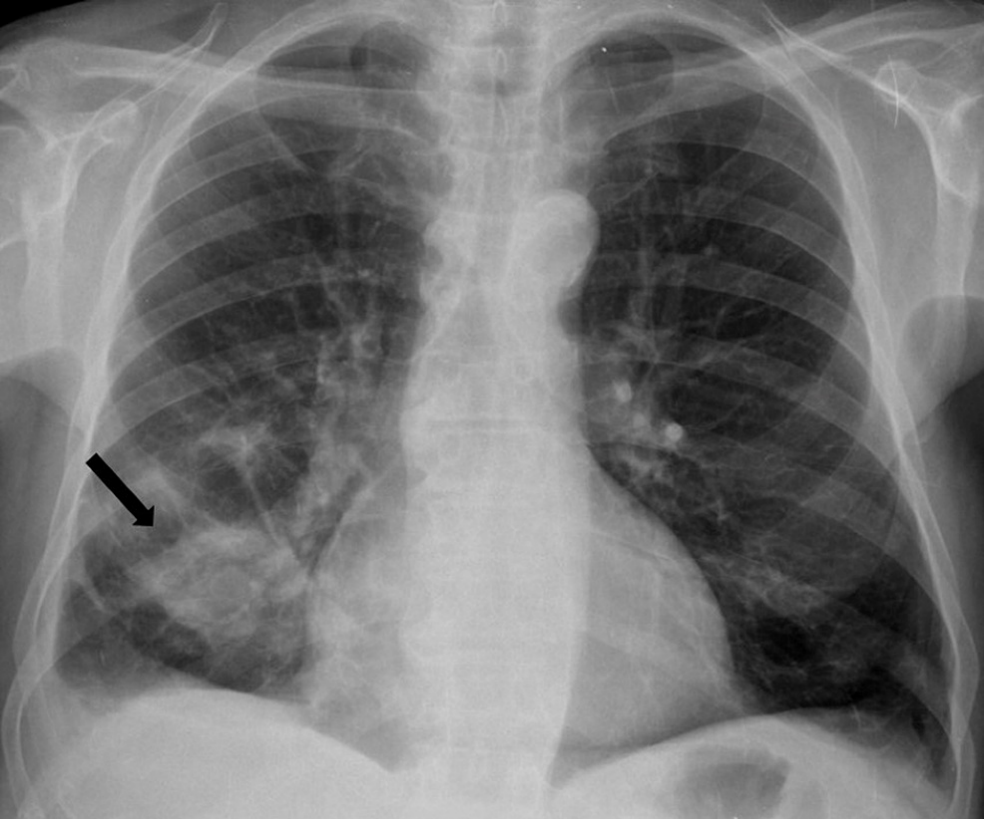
Chest X-ray obtained three weeks after the first observation, revealing a new condensation in the inferior lobe of the right lung (black arrow).
